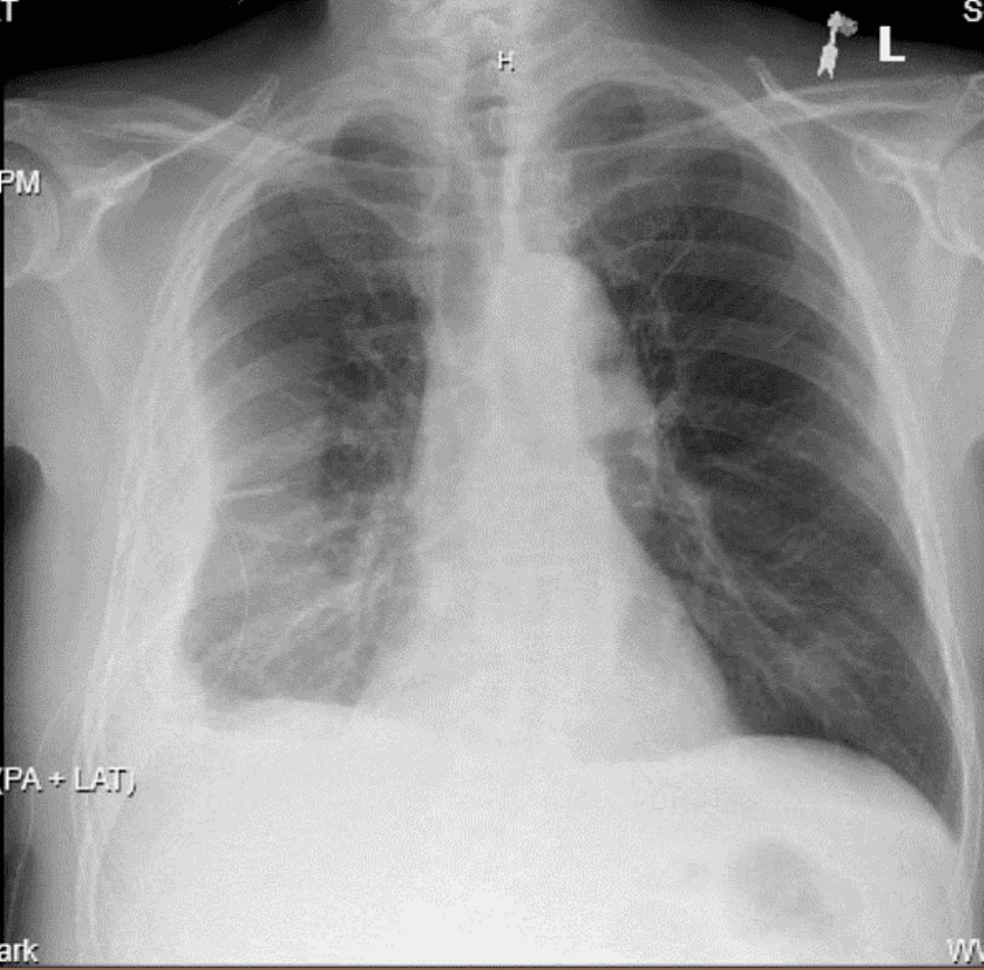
AP CXR one month prior to hospitalization shows a small partially loculated right pleural effusion with the chest tube in the appropriate position and hazy atelectasis versus infiltrate in the mid to lower right lung AP: anteroposterior; CXR: chest X-ray
